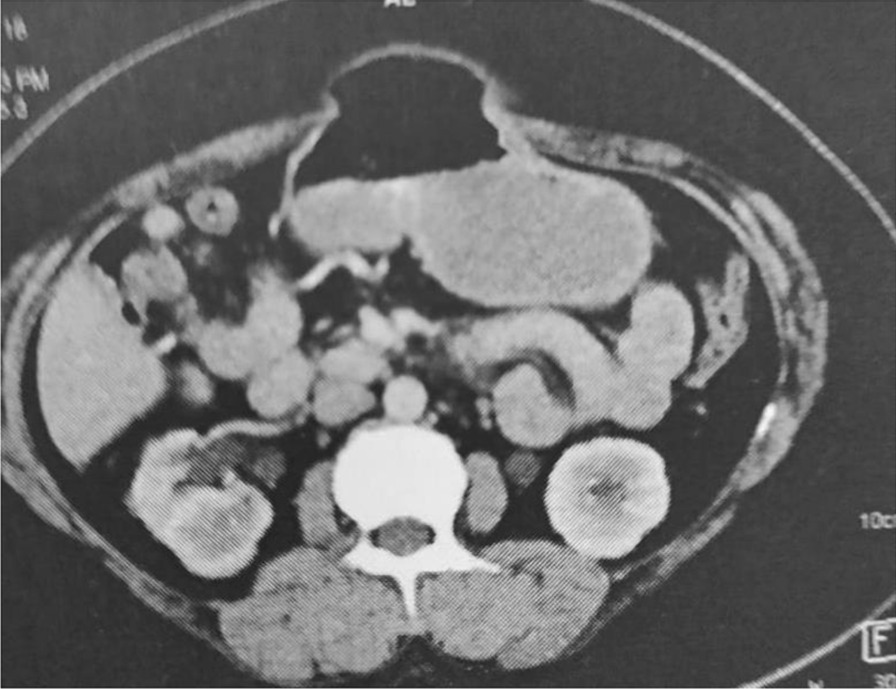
CT of abdomen shows burst abdomen with absence of intra-abdominal abscess
